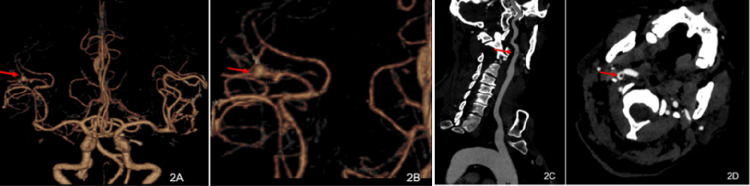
(A-B) Head and neck computed tomography angiography on June 1 indicating multiple aneurysms in the M3 segment of the right middle cerebral artery (arrow indicated). (C-D) Head and neck computed tomography angiography on June 1 indicating mural thrombus formation in the C1 segment of the right internal carotid artery (arrow indicated)
